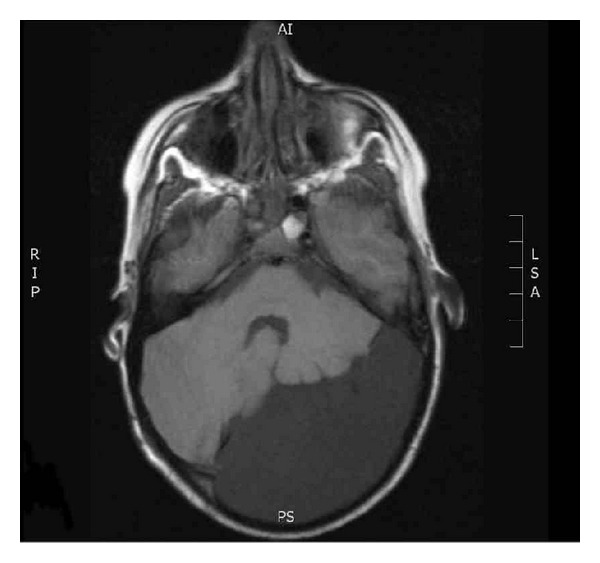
Axial MRI Head scans showing the presence of a large posterior fossa arachnoid cyst, this is predominantly left sided, causing mild midline shift and some distortion of the ventricular system. There is no hydrocephalus.
